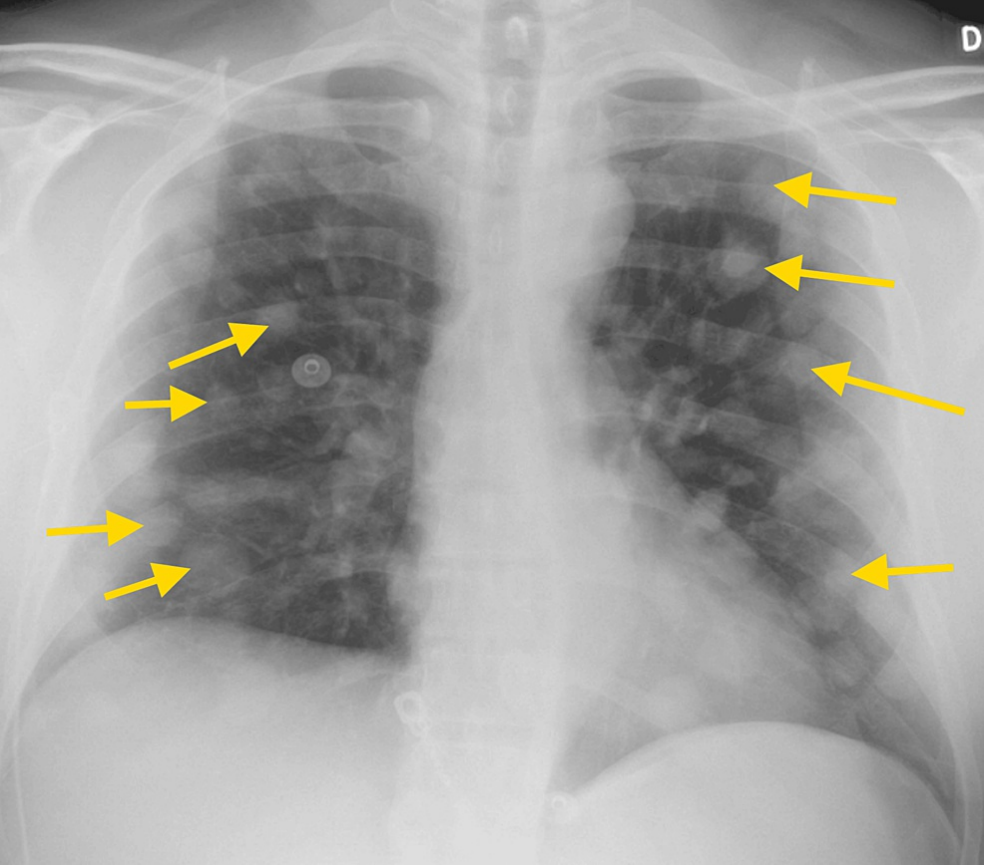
AP chest X-ray of the patient showing numerous pulmonary nodules Several of the pulmonary nodules are pointed out with yellow arrows. AP: anteroposterior
